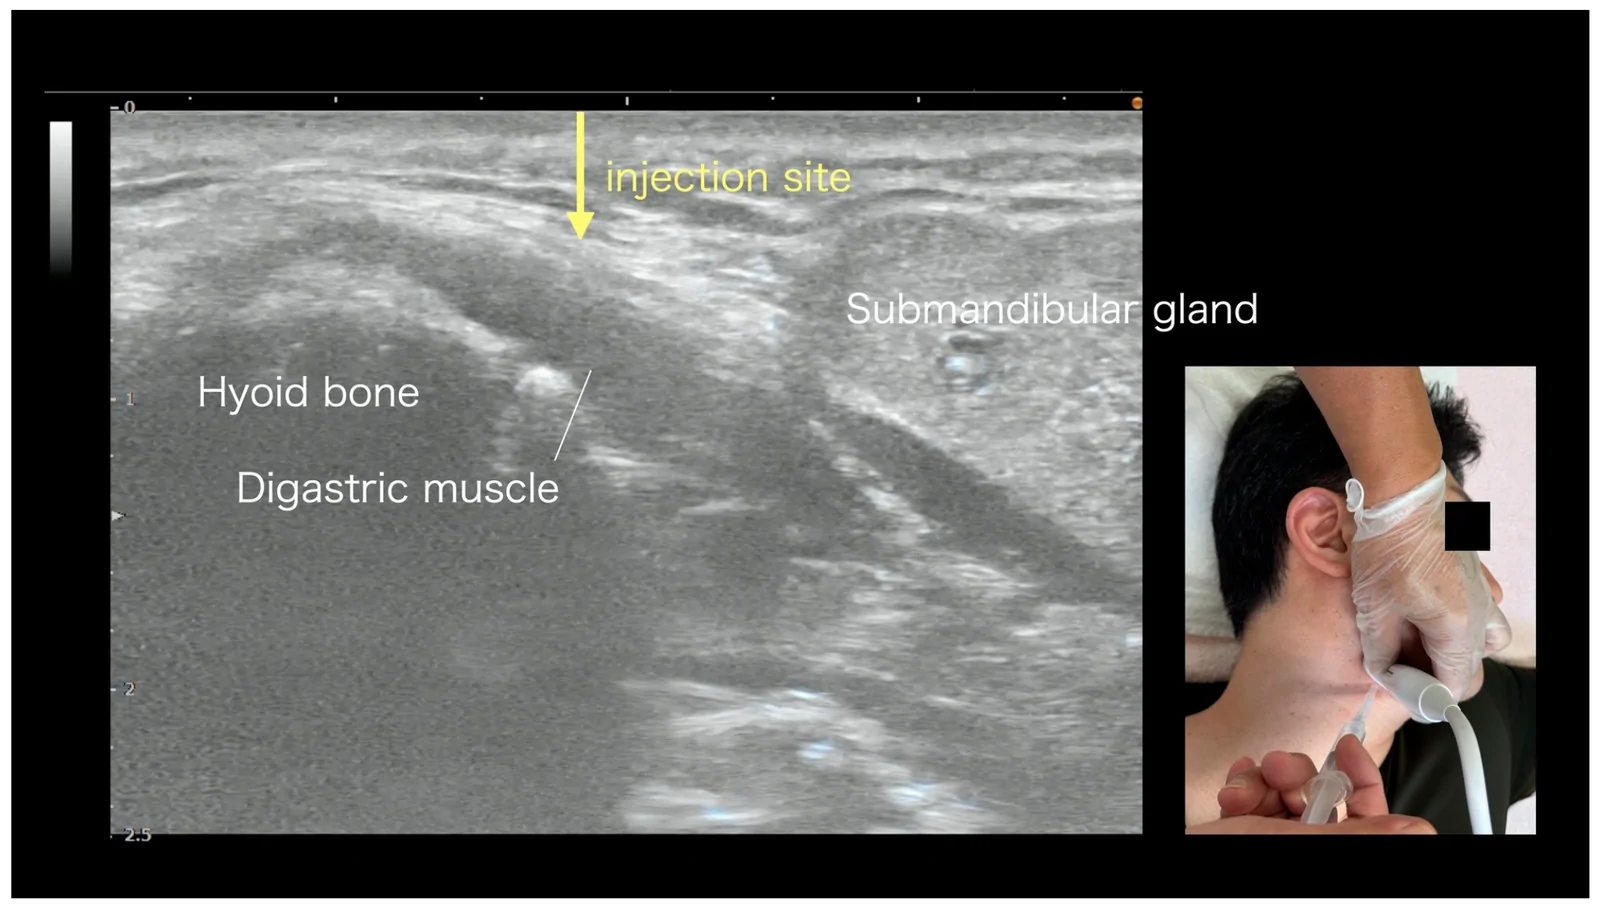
Digastric muscle US-FHR (POINT 8). Ultrasound image: Hyoid bone, digastric muscle, and submandibular gland are identified. The yellow arrow (injection site) indicates the fascia adjacent to the digastric muscle. With a 27 G 38 mm needle, 1–2 mL of saline is injected via the out-of-plane approach. The inset (bottom right) shows the patient position and probe placement.
